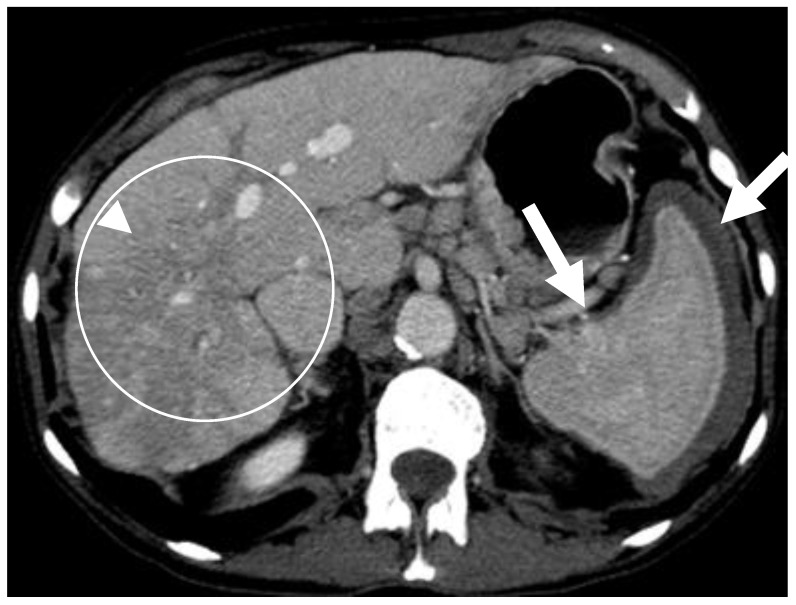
A 59-year-old lady with a 20-year history of systemic mastocytosis. Axial contrast-enhanced CT demonstrates hepatomegaly and a large heterogeneous mass with areas of arterial enhancement and heterogeneous hypoattenuating apperance on portal venous phase (circled), increased retraction of the hepatic capsule related to volume loss (arrowhead), and an abnormal heterogenous appearance of the spleen with surronding ring of hypoattenuating soft tissue (white arrows).
